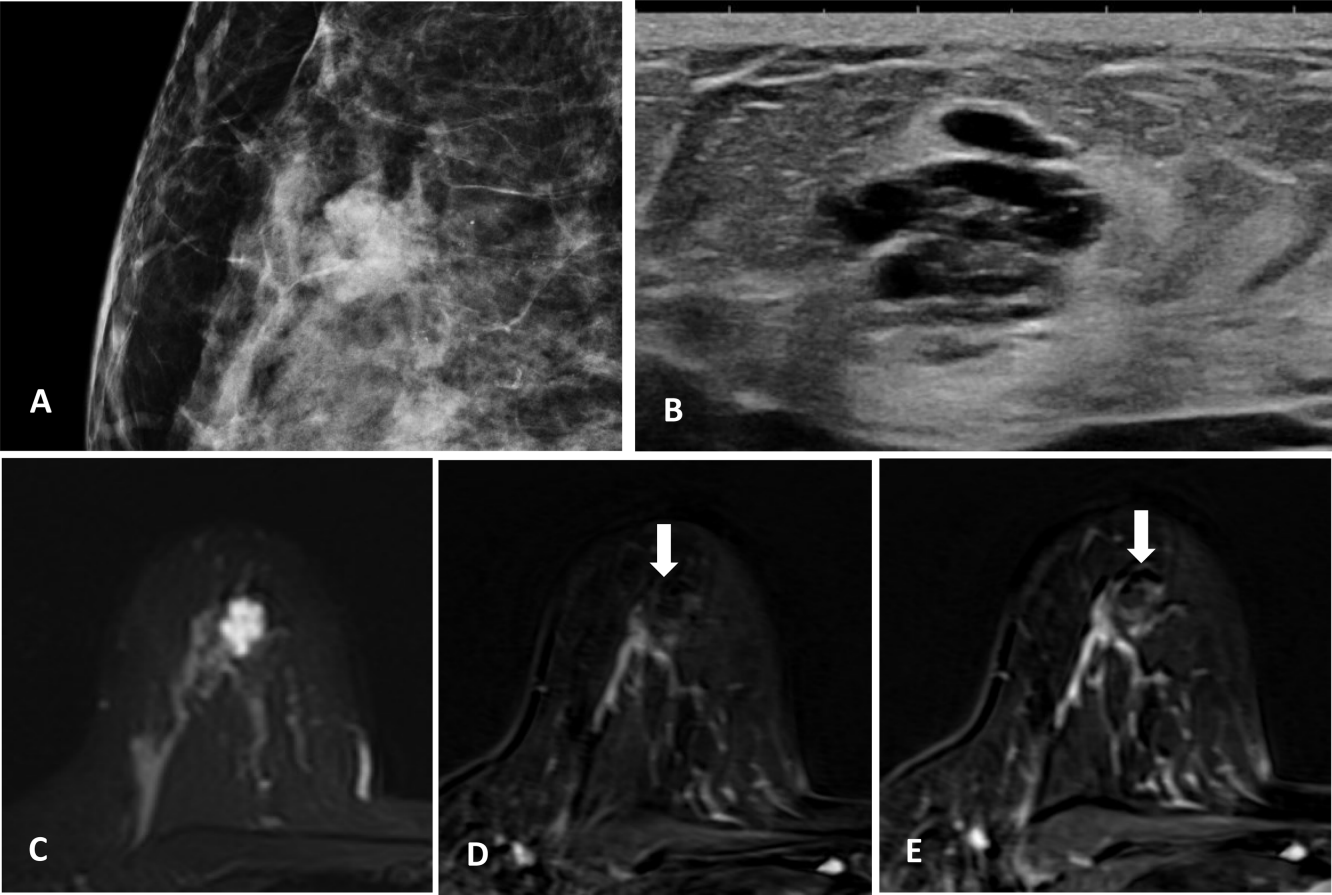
(A) Irregular high-density mass with microlobulated margins on mammography, no calcification within the lesion. (B) Heterogeneous irregular mass with circumscribed margins on ultrasound. (C) MR T2-weighted sequence, the lesion is lobular and shows high signal intensity. (D, E) MR-enhanced T1-weighted sequence (immediately after contrast injection and about 6 min delay), the lesion shows persistent slight enhancement at the periphery with no internal enhancement.
